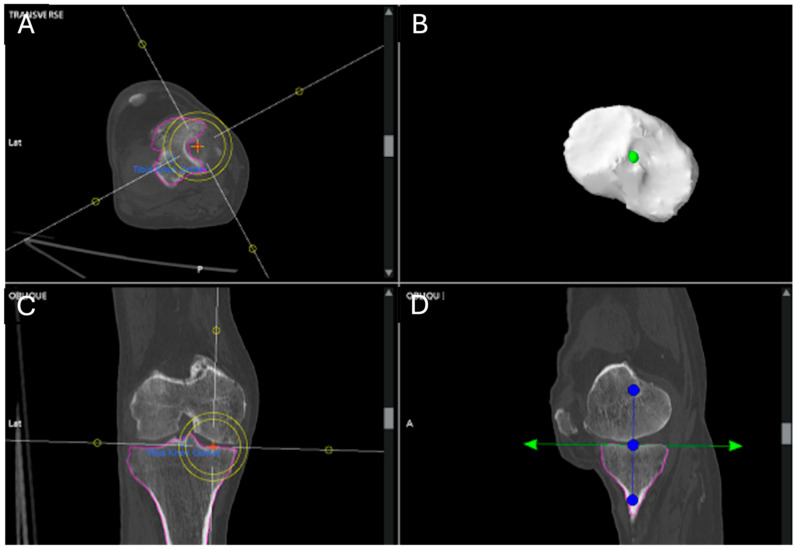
3D CT-based measurement of the posterior tibial slope (PTS) using the robotic planning software. The screen displays four corresponding views used to define the anatomical landmarks and axes: (A) transverse view, (B) 3D bone model, (C) coronal view, and (D) sagittal view. In the sagittal view (D), the medial posterior tibial slope (MPTS) is determined automatically. The blue dots define the tibial mechanical axis, and the green line represents the perpendicular reference axis used to calculate the slope.
